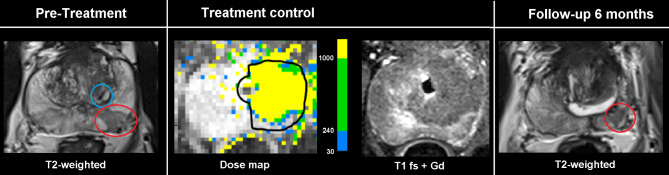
Calcification and treatment failure. Images of a 55-year-old patient with localized PCa in the left peripheral posterior zone (Gleason 3 + 4) undergoing a TULSA hemiablation. A small linear hypointense calcification is depicted in the pre-treatment T2-weighted images (blue circle). The tumor is marked by a red circle. A clear undertreatment distal from the calcification within the segmented tissue (black line) is visible in the dose map and viable tissue is shown in the T1-weighted image after contrast administration. The residual tumor is verified in the T2-weighted follow-up examination after 6 months (red circle)
